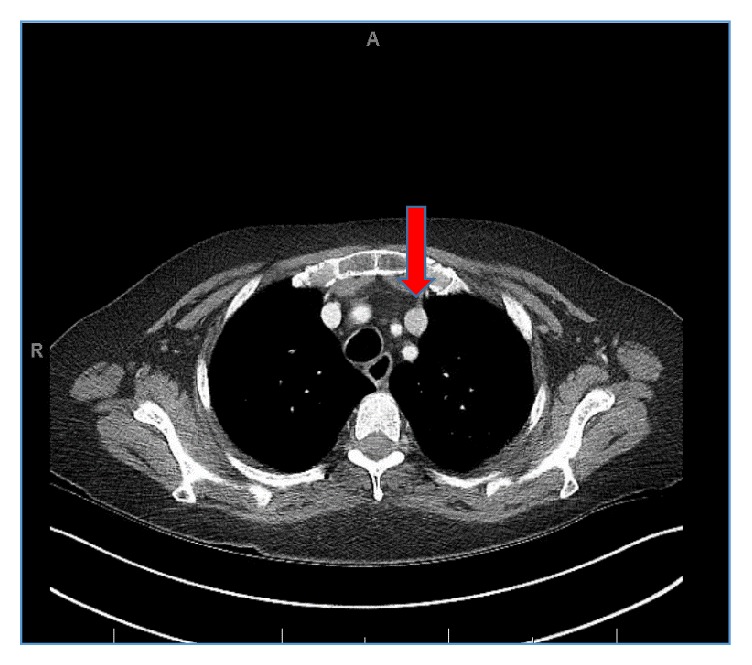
CT thorax visualizing persistent left superior vena cava.
